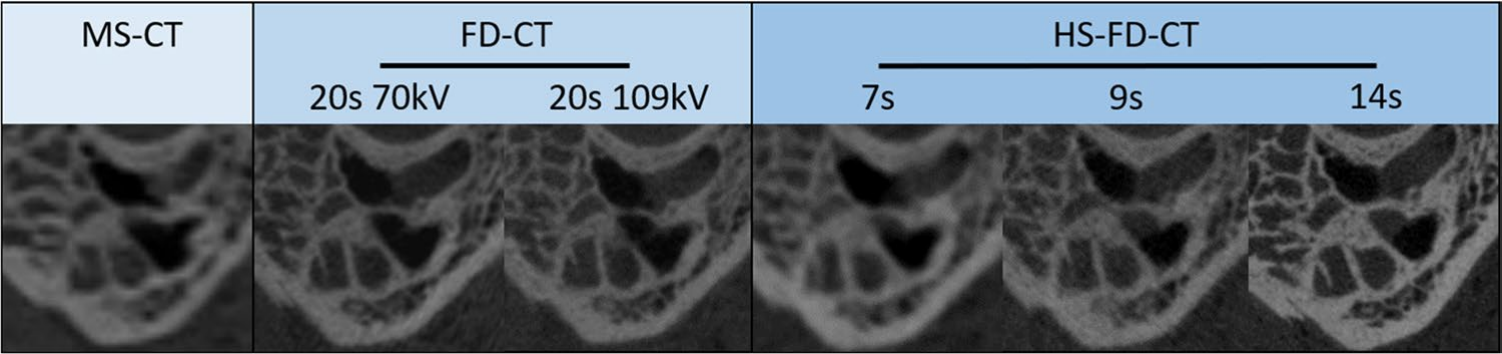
MS-CT, FD-CT, and HS-FD-CT MRPs of the mastoidal cells comparing different scanning protocols
